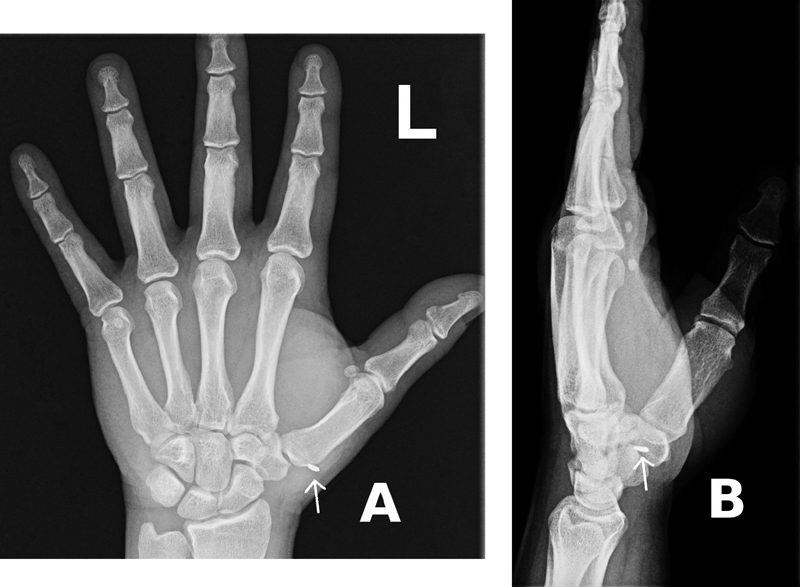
Radiolucent foreign body was detected on simple X-rays. (A) Anteroposterior and (B) profile of the patient's left hand (white arrows).
